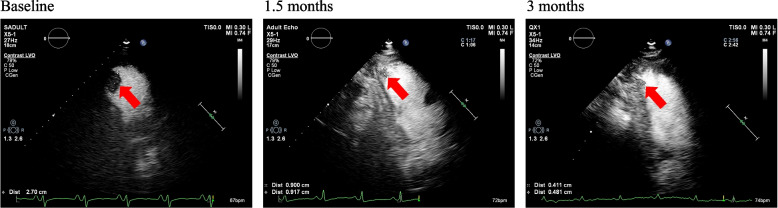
Patient example of CE images. The thrombus is persistent at 1.5 months and 3 months, compared to LV thrombus (red arrows) at baseline. LV, left ventricular; CE, contrast-enhanced echocardiography
